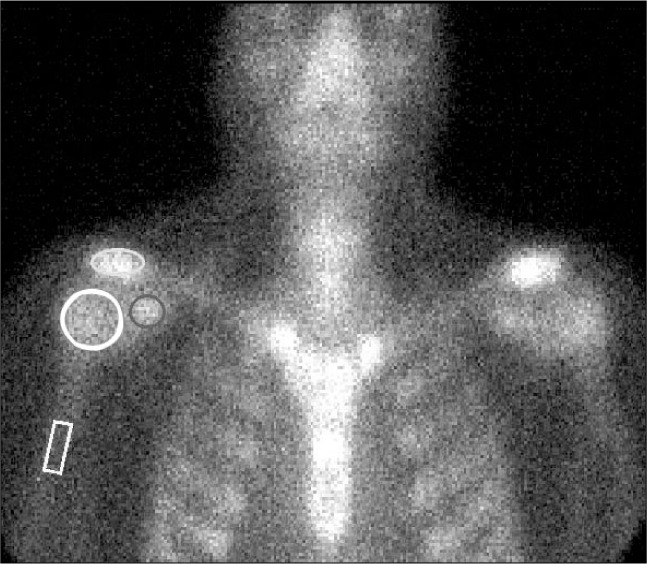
Regions of interest (ROIs) for the shoulders were outlined for the acromioclavicular joints, coracoid processes and humeral heads, and the humeral shaft was used as the reference in the analysis with three-phase bone scintigraphy.
